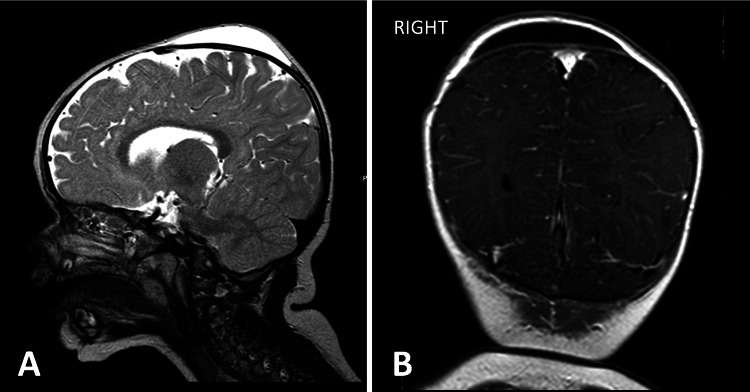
Brain MRI showing presence of biparietoccipital subaponeurotic fluid collection with greater expression on the right, without evidence of skull fracture or intracranial abnormality. (A) Sagittal image. (B) Coronal image.
